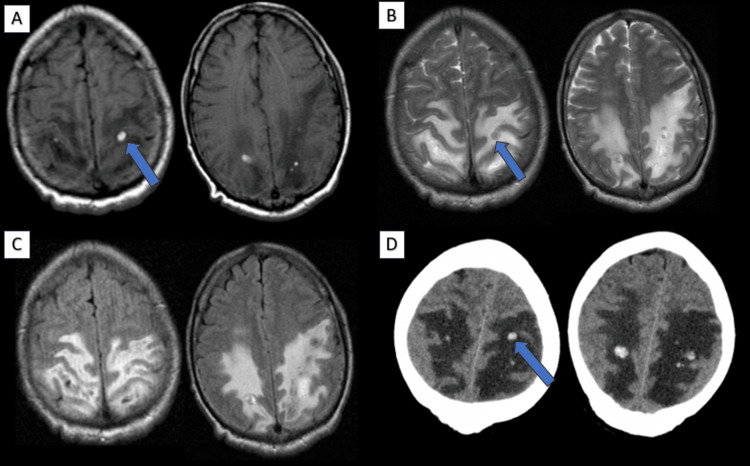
Axial T1W (A), T2W (B), and FLAIR (C) brain MRI and axial NECT of the brain (D) images. A 36-year-old male, a post-renal transplantation case on tacrolimus, presented with headache, seizures, and left upper and lower limb weakness. Brain MRI images showed hemorrhagic foci (blue arrow) with surrounding edema in the bilateral frontoparietal lobes. This patient had a residual focal neurological deficit in the form of left hemiplegia on follow-up. T1W: T1-weighted; T2W: T2-weighted; FLAIR: fluid-attenuated inversion recovery; NECT: non-enhanced computed tomography.
